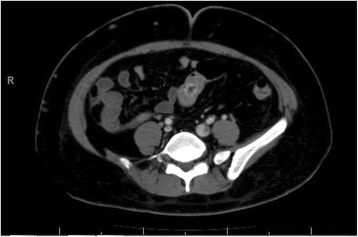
Computed tomography scan revealing the extension of the clot to the common iliac veins.
